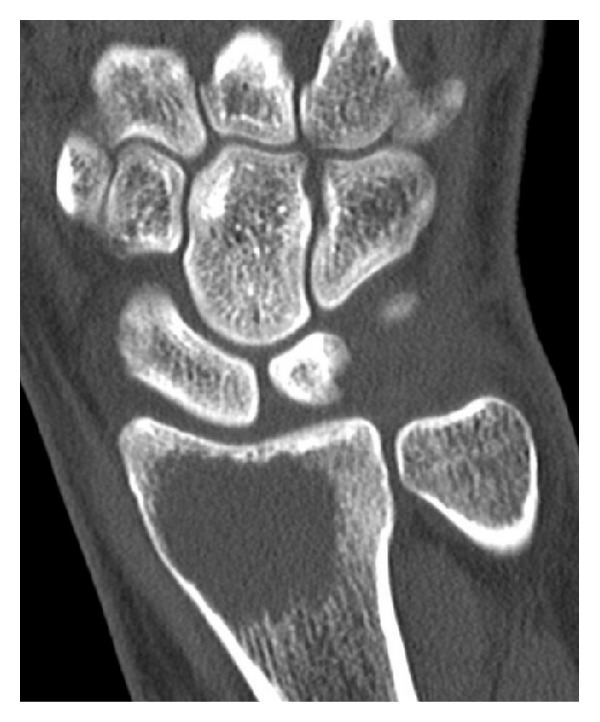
Computed tomography of the right wrist (coronal section): the extension of the lytic lesion in the subchondral region of the distal radius is well recognized.
